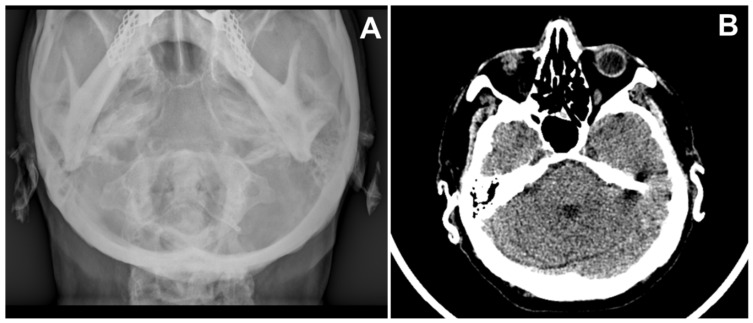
(A) X-ray of the skull showing opacification of both helices; (B) Computerized tomography of skull reveals calcification of right and left pinna.
